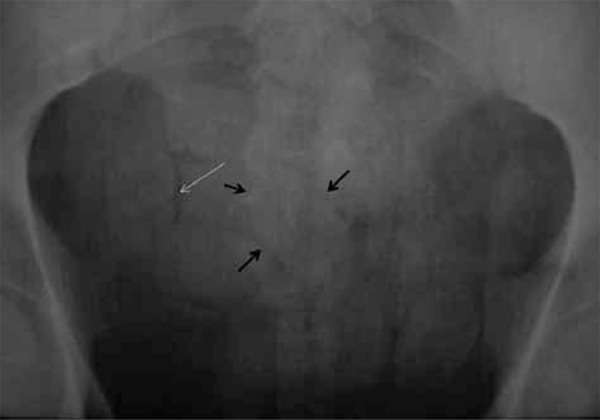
Pelvic x-ray demonstrates multiple radiopaque foreign bodies. Double-condom sign (black arrows), as air trapped between layers of latex and rosette-like appearance (white arrow), made by air trapped in condom knot are depicted.
